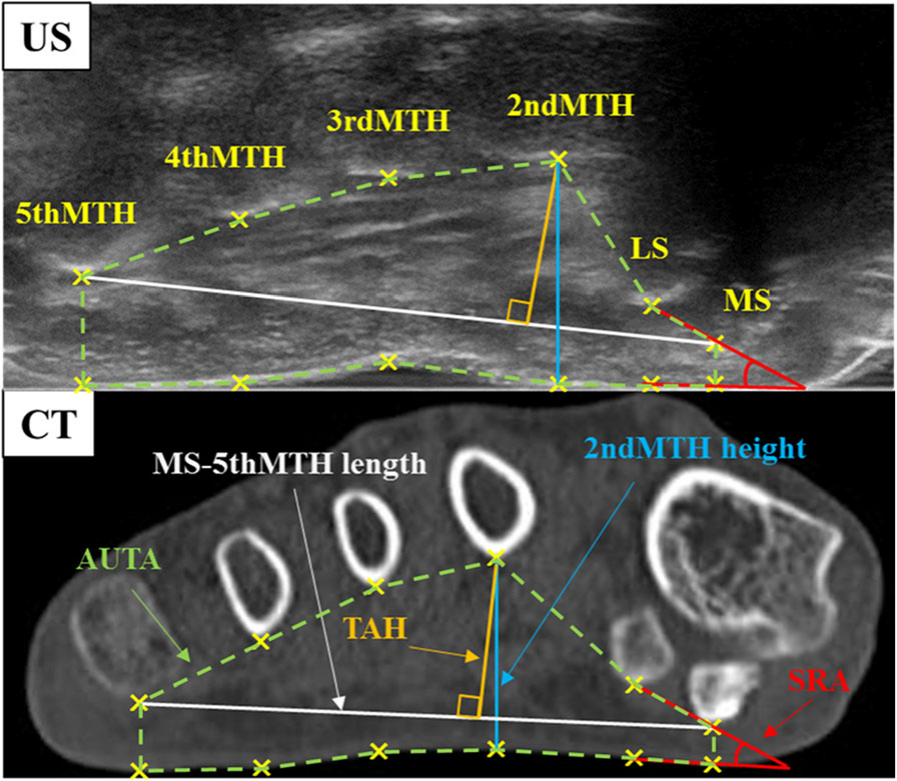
Forefoot structure images in the coronal plane obtained from ultrasonography (US) and computed tomography (CT). The six points of the bones, medial sesamoid bone (MS), lateral sesamoid bone (LS), 2nd metatarsal head (MTH), 3rdMTH, 4thMTH and 5thMTH, were marked. In addition to these six bone points, six plantar points were plotted under each bone point, and all parameters were measured. The length of the *yellow line* indicates the transverse arch height (TAH). The length of the *blue line* indicates the 2ndMTH height. The length of the *white line* indicates the length between the medial sesamoid bone and 5th metatarsal head (MS-5thMTH length). The angle composed of the red lines indicates the sesamoid rotation angle (SRA). The area surrounded by the *green dashed* line indicates the area under the transverse arch (AUTA). As the ultrasound waves cannot penetrate bones, the ultrasonogram of the forefoot demonstrates only the plantar surfaces of sesamoid bones and the 2nd through 5th MTHs
